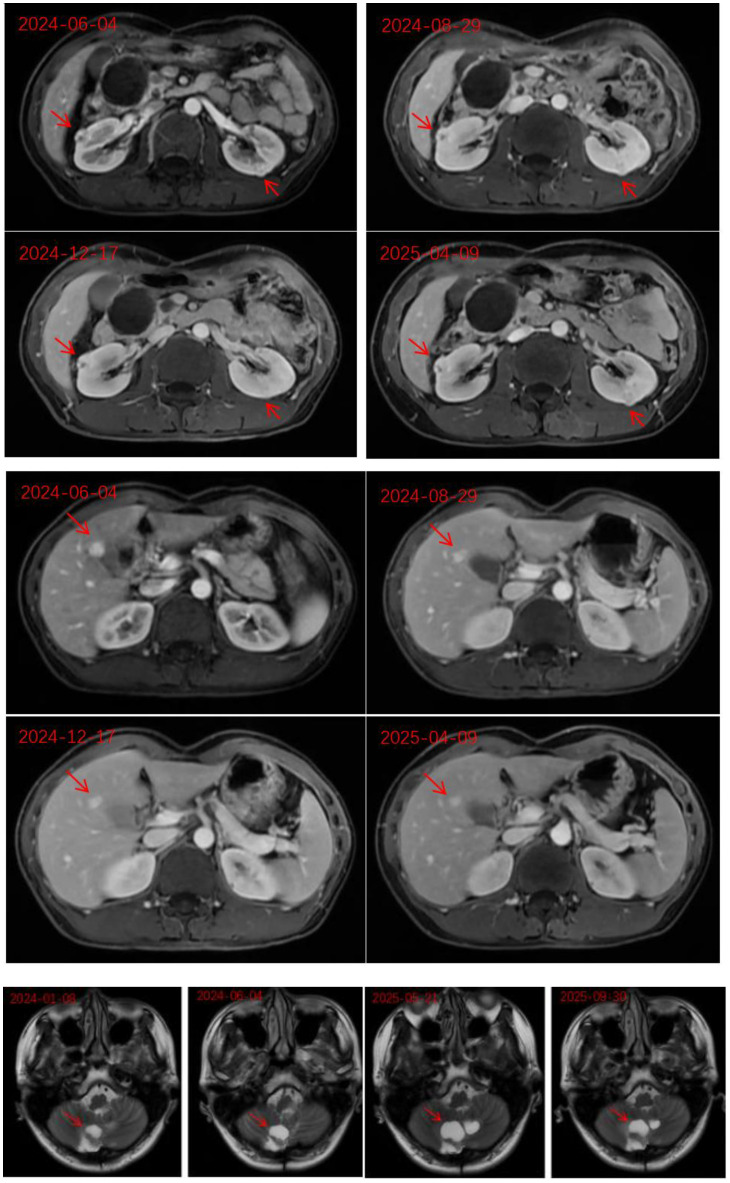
Abdominal and pelvic contrast-enhanced MRI of the abdominal and pelvic cavity indicates two highly enhanced lesions in both kidneys and abnormally enhanced lesion in the left hepatic lobe, with follow-up showing the lesions to be similar to previous assessments; Cranial MRI indicates multiple abnormal signals in the cerebellum, medulla oblongata, and fourth ventricle, with follow-up suggesting progression of the cranial lesions which later reduced in size.
